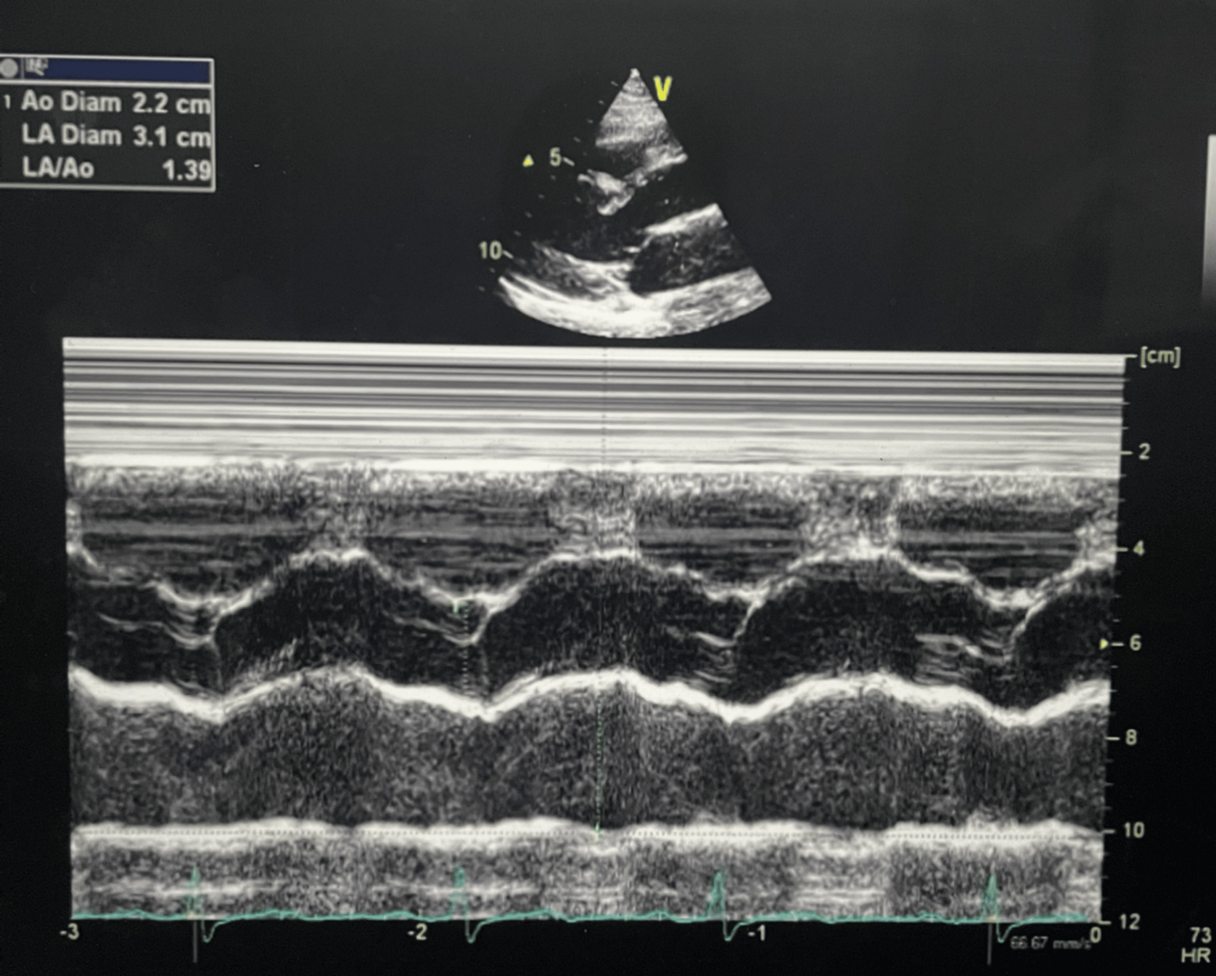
M-mode echocardiogram showing normal aortic root diameter (2.2 cm) and normal left atrial diameter (3.1 cm).
